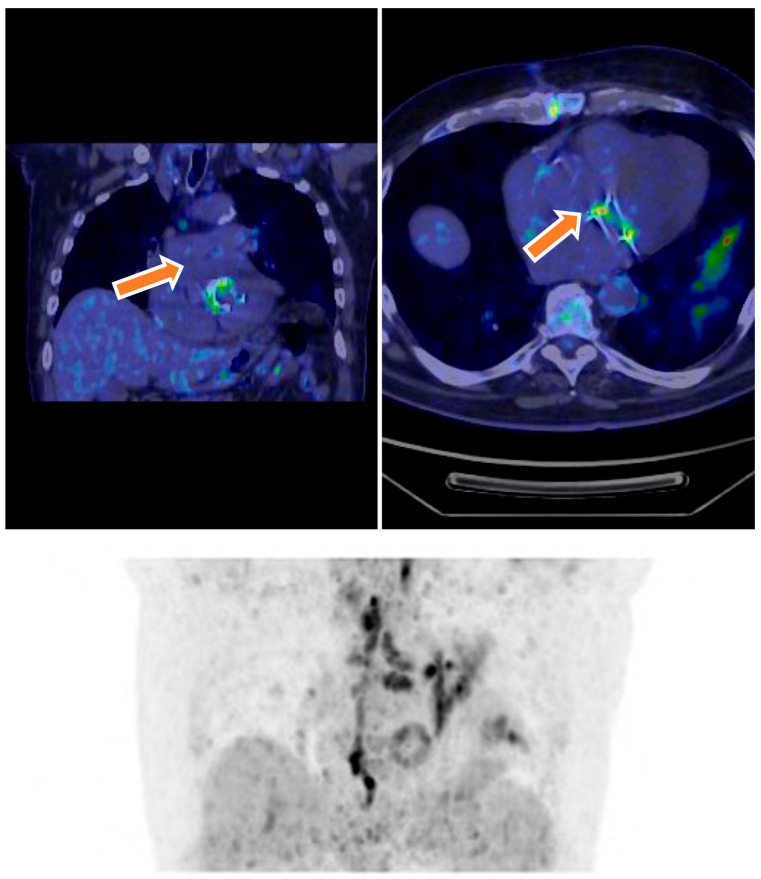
Transverse and coronal ^18^F-FDG PET/CT images show an infected prosthetic mitral valve. Culture after valve removal was positive for *Staphylococcus aureus.* ^18^F-FDG PET/CT image demonstrating abnormal metabolic activity around a prosthetic mitral valve lead and adjacent valve in a 75-year-old female. The PET/CT findings defined the presence of device-related infection and contributed to the classification of definite endocarditis.
